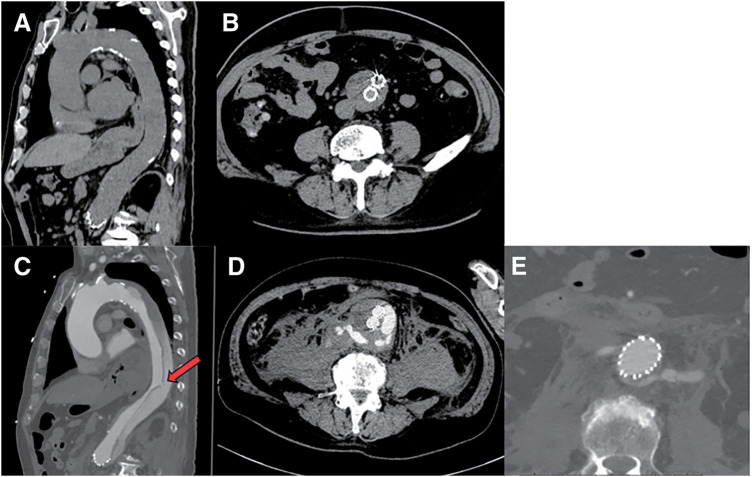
Fig. 1 Serial CT findings before and after onset of type B aortic dissection. (A, B) CT at the initial emergency department visit. The AAA showed a tendency toward reduction, and no clear findings suggestive of aortic dissection were observed. (C) CT at representation demonstrated an acute Stanford type B aortic dissection with a large primary entry tear located in the descending thoracic aorta (arrow). The false lumen extended from the origin of the left subclavian artery to the infrarenal AAA. (D) A retroperitoneal hematoma adjacent to the AAA indicated rupture of the false lumen within the aneurysm. (E) A suspected re-entry tear was identified at the level of the left renal artery. CT: computed tomography; AAA: abdominal aortic aneurysm
